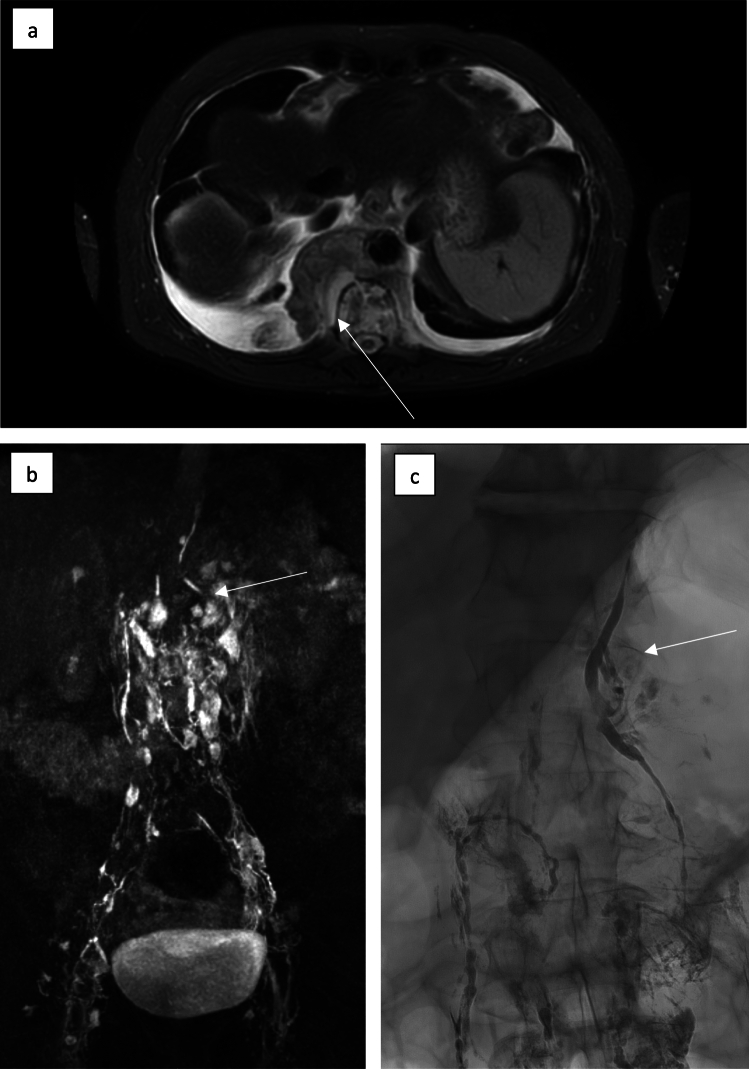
MR lymphangiography of a 56-year-old patient with extensive retrocural lymphoma manifestation (white arrow) (a). After nodal contrast application KM ascension via enhancement of pelvic and retroperitoneal lymphatic vessels is visible; in the upper abdomen the lower part of the thoracic duct can be seen. There is no further enhancement of the thoracic duct above the lymphoma mass corresponding to lymphatic obstruction (white arrow) (b). X-ray lymphangiography  corroborated  obstructive lymphatic drainage disorder at the level of the retrocrural lymphatic mass (white arrow) and consecutive chylolymphatic reflux in the upper abdomen (c)
